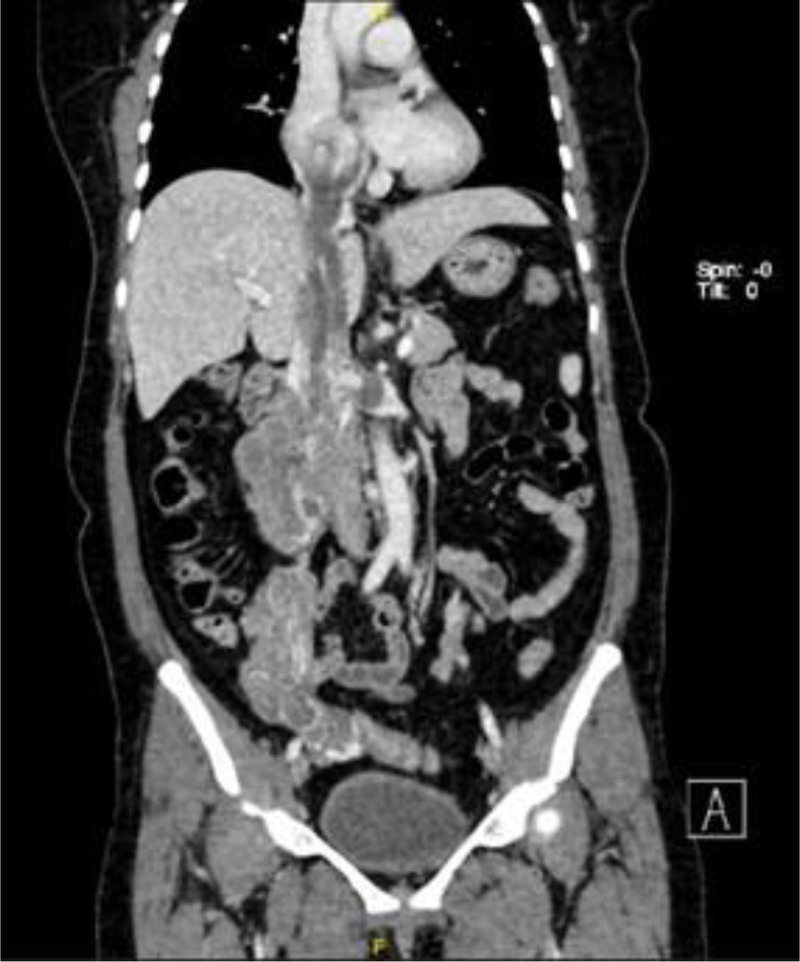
Lower limb deep venous CTV of case 2. CTV showed an uneven mass stretching from the right ovarian veins to the right atrium, supporting IVL. CTV = computed tomography venography, IVL = intravenous leiomyomatosis.
